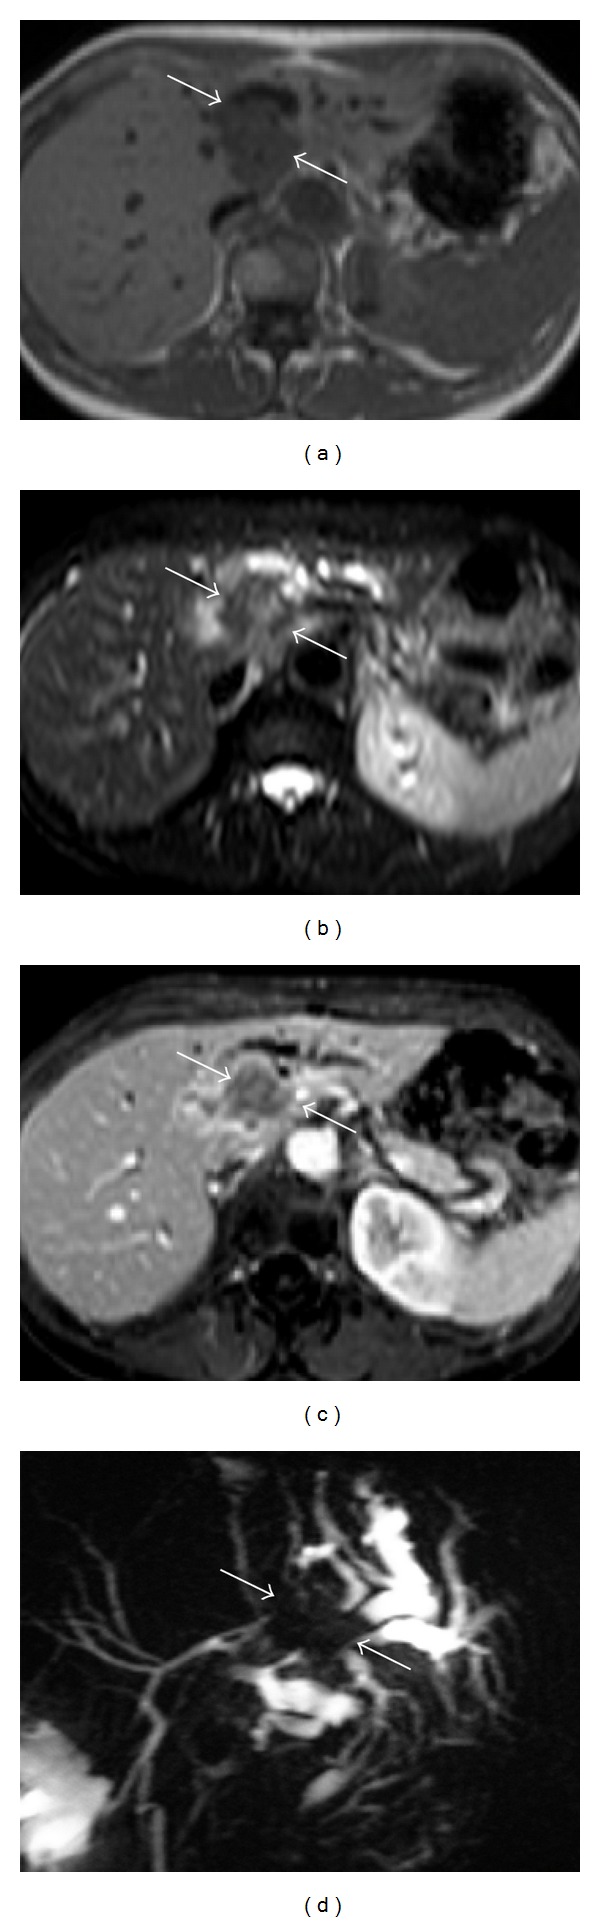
This figure demonstrates a mass lesion located in the left lobe of the liver close to hilus (a) hypointense on T1-weighted MR image, (b) heterogenous hyperintense on T2-weighted image, and (c) heterogenous contrast enhancement on contrast-enhanced fat-saturated T1-weighted image. (d) MRCP image demonstrates the mass that originated from left main bile duct and causes significant bile duct dilatation at the periphery of the liver (arrows). The lesion was considered to be a cholangiocarcinoma with MRI and MRCP findings and also confirmed surgically.
